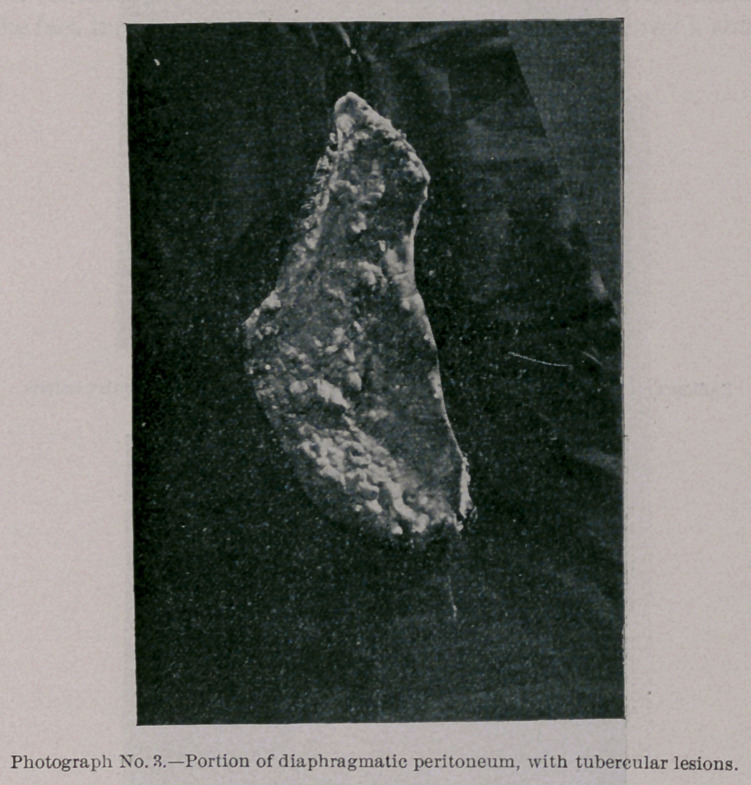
Radiograph No. 3. f6:
